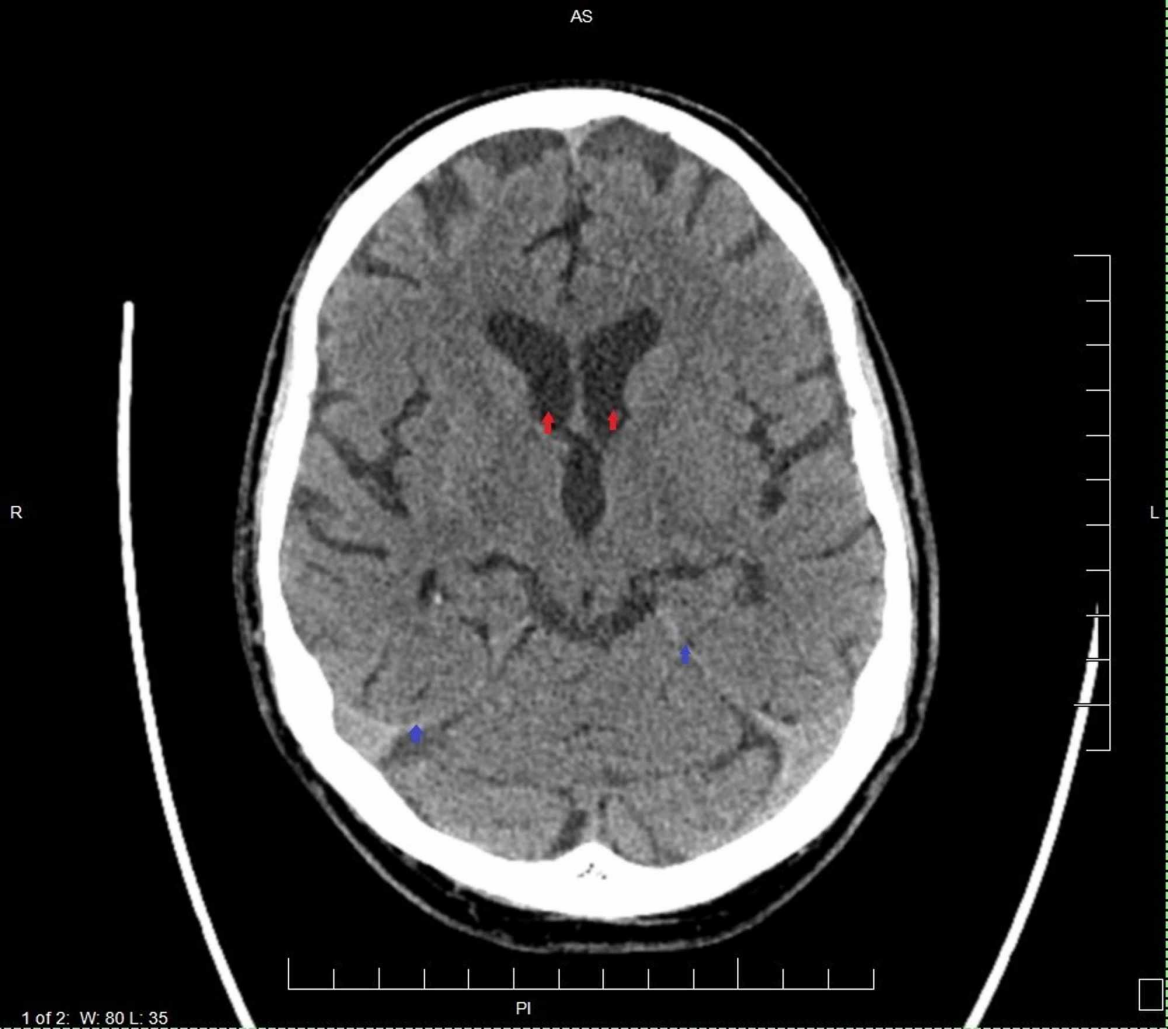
CT scan of the head without contrast. The ventricles and extra-axial spaces are normal (red arrows). No hemorrhage, mass, or mass effect. Normal brain parenchyma. Moderate atherosclerotic calcifications (blue arrows) L: Length; W: Width; R: Right; L: Left; AS: Anterior side; P: Posterior
